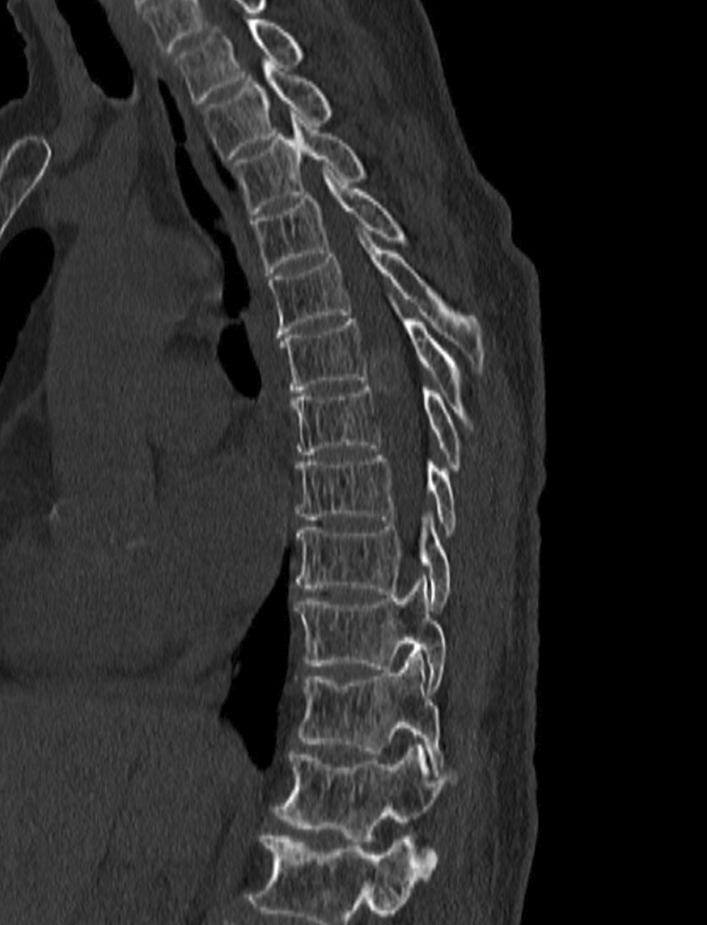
Computed tomography demonstrated a large oval-shaped mass with mild calcification at T6-7 level
